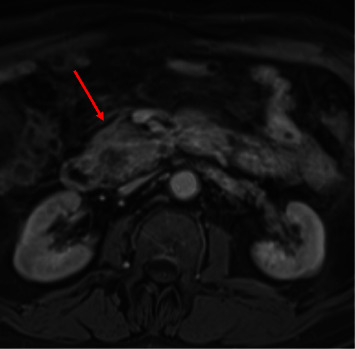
MRI of the abdomen showing ill-defined mass in the inferior pancreatic head/uncinate process with small hypervascular component and large hypovascular component measuring 1.9 × 2.0 cm. There is adjacent side branch ectasia but no dilatation of the main pancreatic duct.
